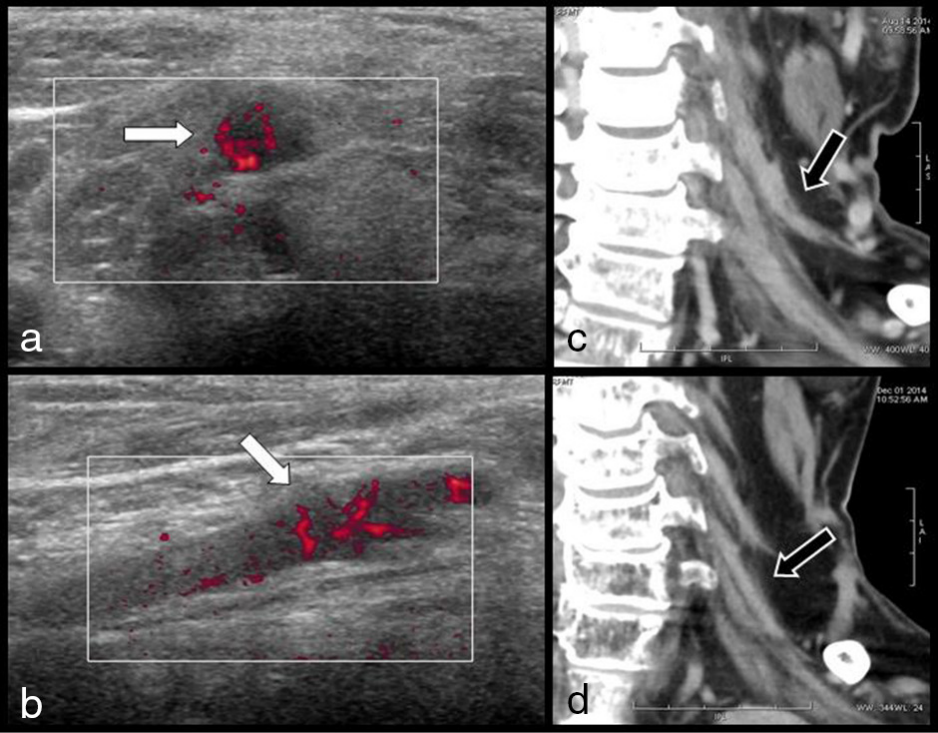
Transverse (a) and longitudinal (b) ultrasound views of the most swollen sixth root. The root is hypoechoic, thickened with irregular and hazy outlines (white arrow). Colour Doppler ultrasound reveals rich anarchic vascularization of the pathologic root. Coronal multidetector CT reconstructions before (c) and 3 months after (d) radiotherapy show a considerable decrease in the caliber of the roots (black arrow). Only some contrast enhancement persists.
